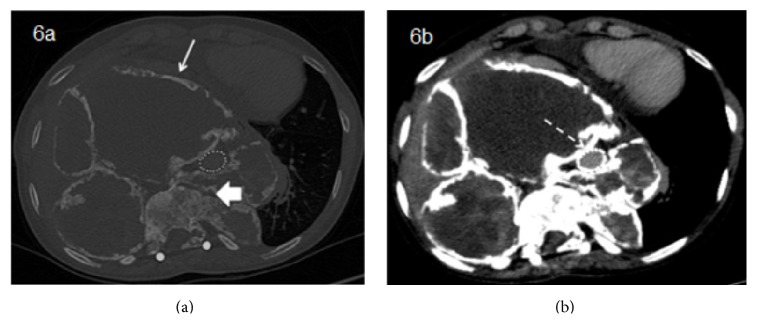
Long-term follow-up CT after continued treatment with denosumab showing more prominent calcification (wide arrow) and reduction of the soft tissue component (narrow arrow). The aortic stent is seen, without extravasation of contrast (dashed arrow).
